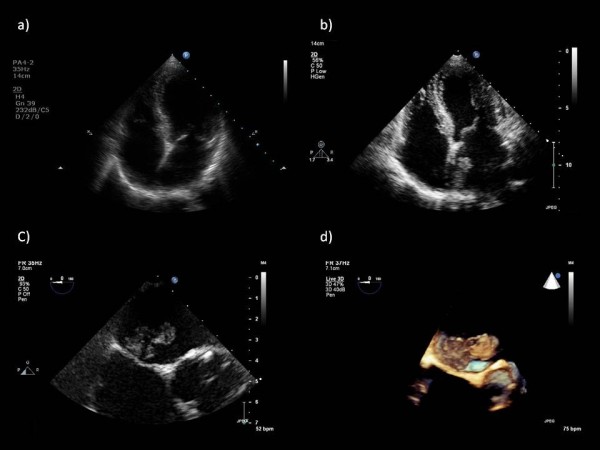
Echocardiography. (A) Transthoracic echocardiogram showing apical four-chamber view and clear left atrium performed a year prior to clinic attendance. (B) Transthoracic echocardiogram showing apical four-chamber view and incidental finding of left atrial myxoma at roof and interatrial septum. (C) Two-dimensional trans-esophageal echocardiogram (TEE) in mid-esophageal four-chamber view, modified and zoomed in on the interatrial septum demonstrating left atrial myxoma attached to the roof of the left atrium and the interatrial septum just above the left ventricular outflow tract and aortic valve. (D) Three-dimensional image of the left atrial myxoma taken from the same TEE mid-esophageal view as in (C). This figure clearly shows the broad peduncle of the myxoma and its attachment to the superior interatrial septum and roof of the left atrium. The three-dimensional TEE assisted the surgeons, by confirming the location of the left atrial myxoma and aiding the planned surgical route.
